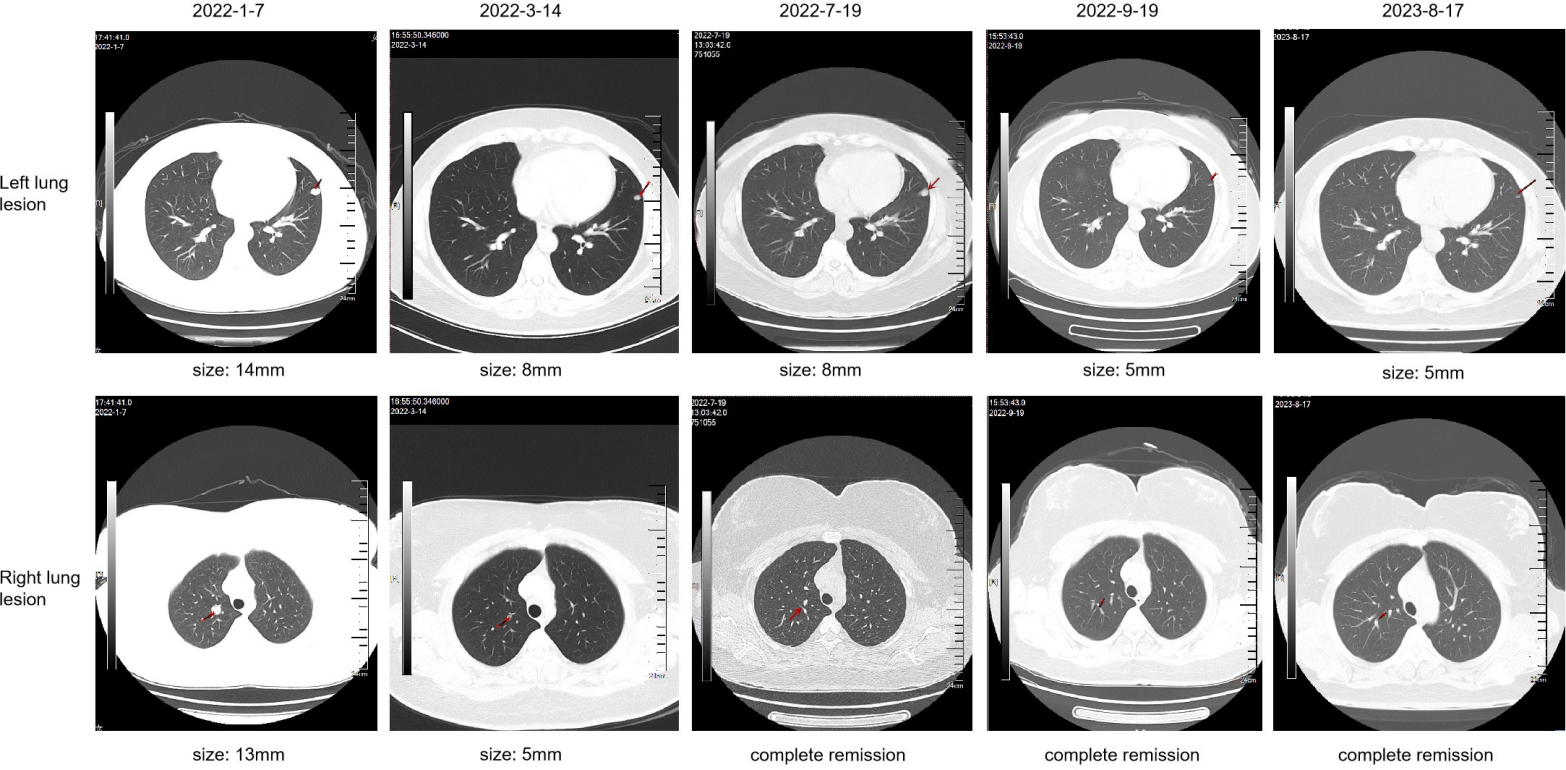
Computed tomography (CT) imaging of lung lesions at critical time point during the treatment course.
